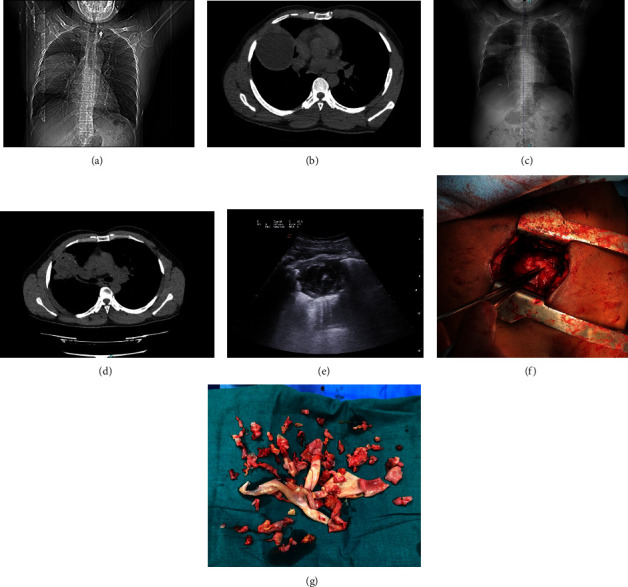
Radiological and surgical findings. (a, b) Thoracic imaging at the time of diagnosis showing a liquid cyst; (c, d) thoracic imaging right before surgery showing a decrease in cystic volume, air within the cyst, and pericystic thickening; (e) ultrasound of the lung cyst carried out during the hospitalization before surgery showing the complex US structure of the cyst, with the “ball of wool sign”; (f, g) intraoperative findings showing the thoracotomic approach and the shreds of pericyst requiring careful dissection.
